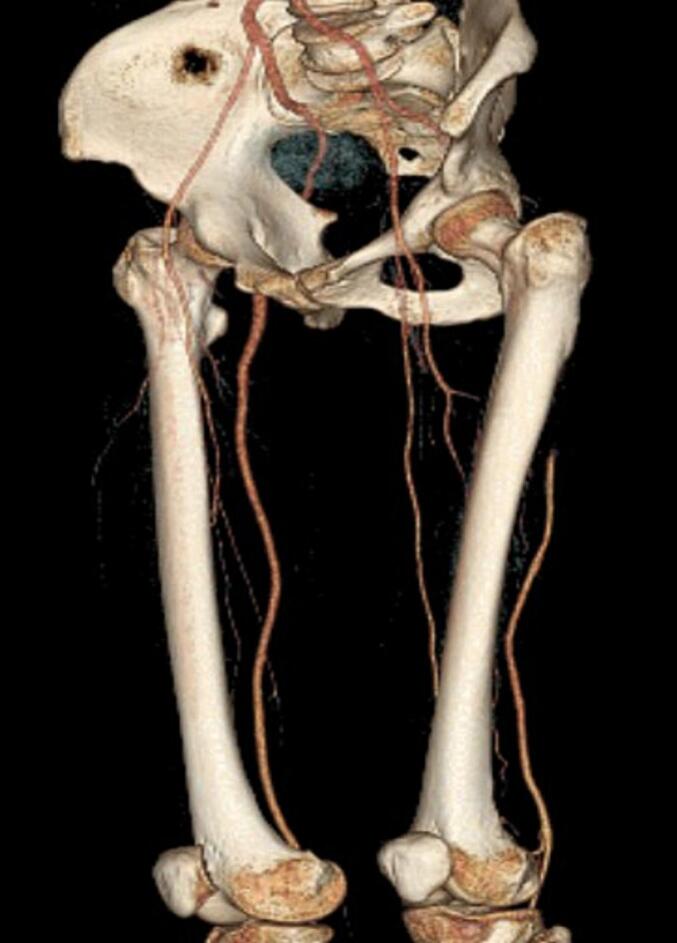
Anterior view of 3D CT reconstruction arteries.
